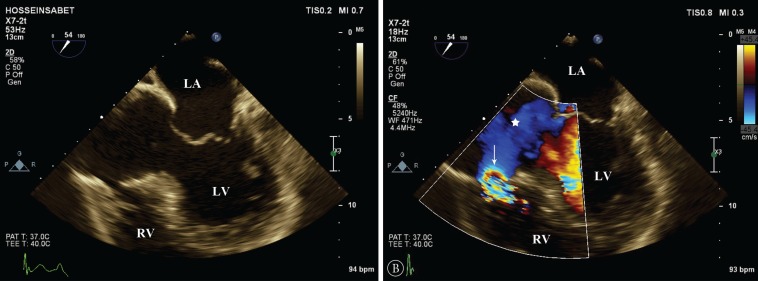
Huge aneurysm (*) in the basal segment of the inferoseptal wall of the left ventricle, protruding into the right ventricle alongside a ventricular septal defect (arrow) in the wall of this aneurysm without (A) and with color Doppler study (B) in transesophageal echocardiography.
